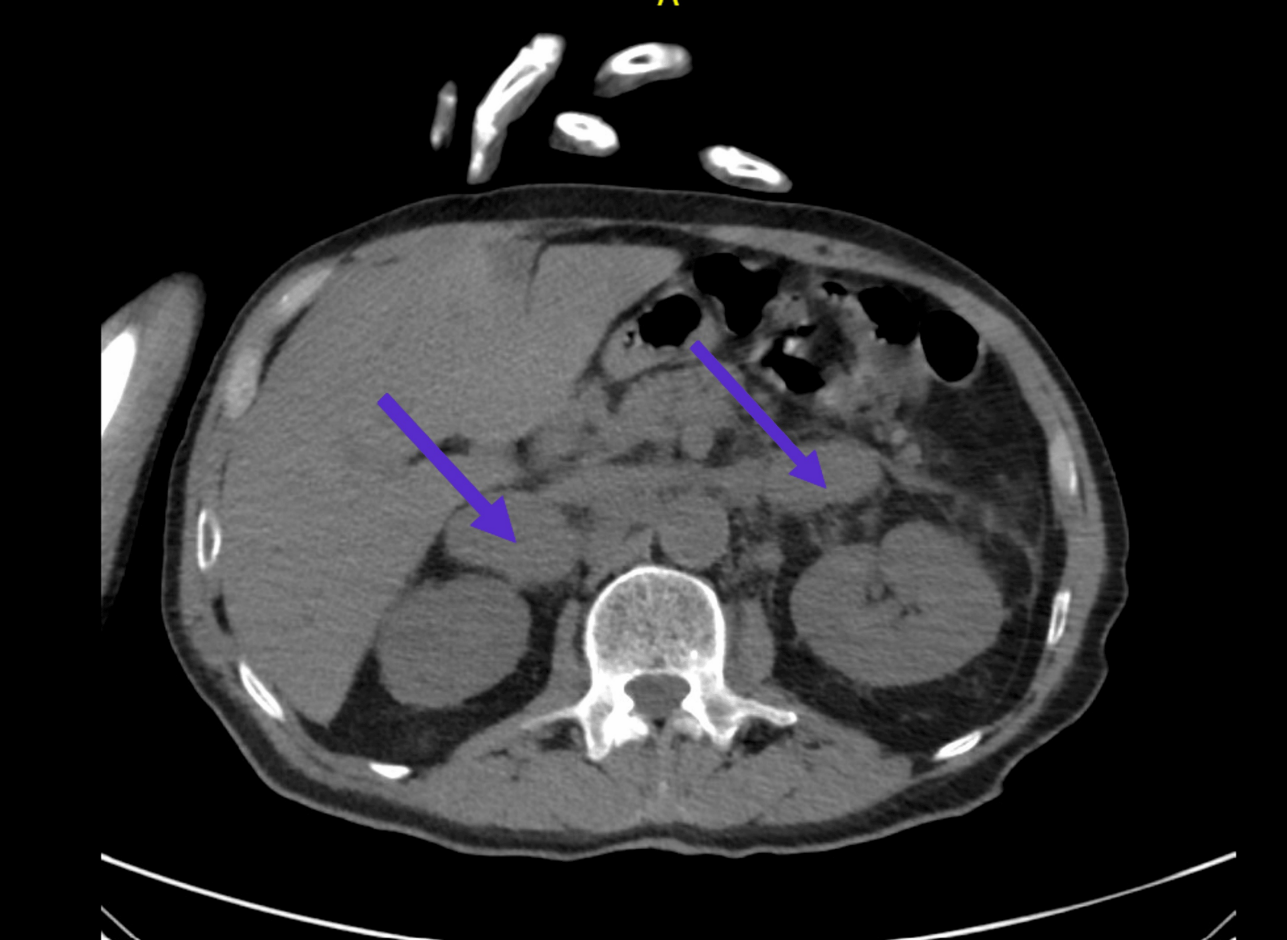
Bilateral adrenal masses (arrows) on non-contrast computed tomography (CT) abdomen and pelvis measuring 5.6 x 3.1 cm (right) and 3.3 x 3.2 cm (left)
